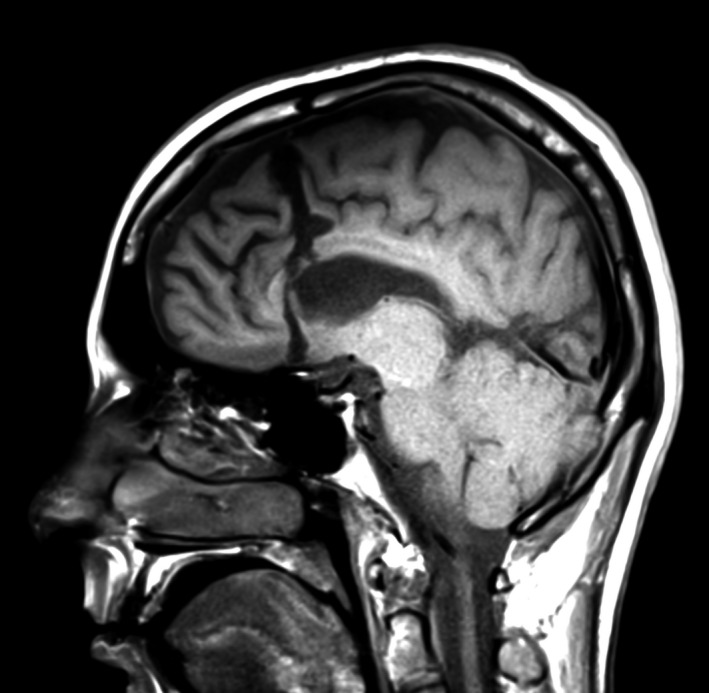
Postsurgical MRI including sagittal T1‐weighted image that demonstrates the frontal disconnection line through the anterior corpus callosum
